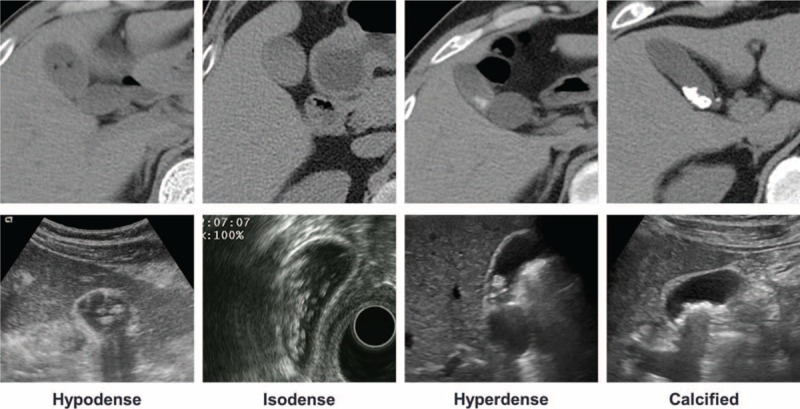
Classification of stone density on CT scan. Images in the above row are representative images of hypodense, isodense, hyperdense, and calcified stones seen on CT scan and those below are corresponding images observed either on abdominal ultrasonography or endoscopic ultrasonography. CT = computed tomography.
